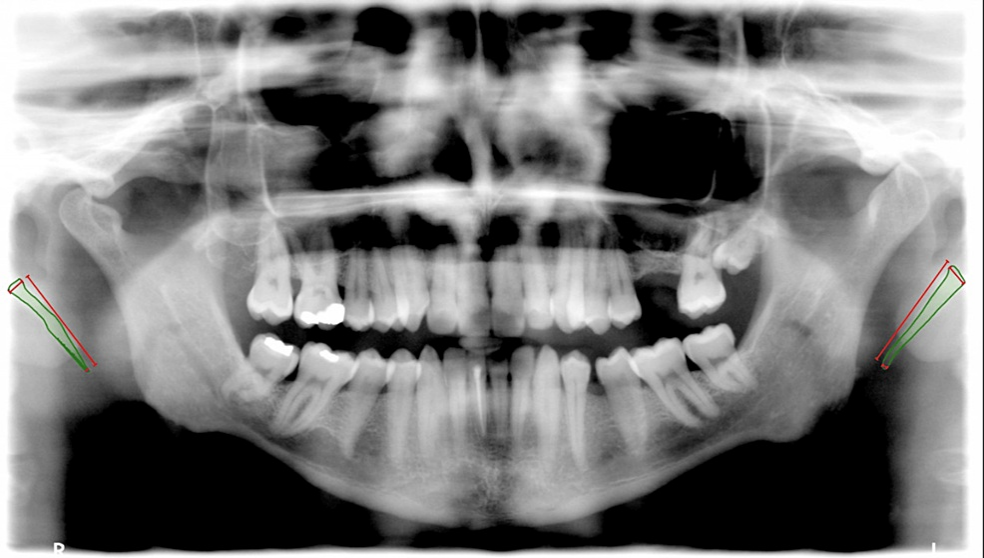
Measurement methods for the styloid process length and diameter at the origin and the end on the panoramic radiograph Green indicates the outline of the styloid process, and red indicates the length and the diameter at the origin and end of the styloid process.
